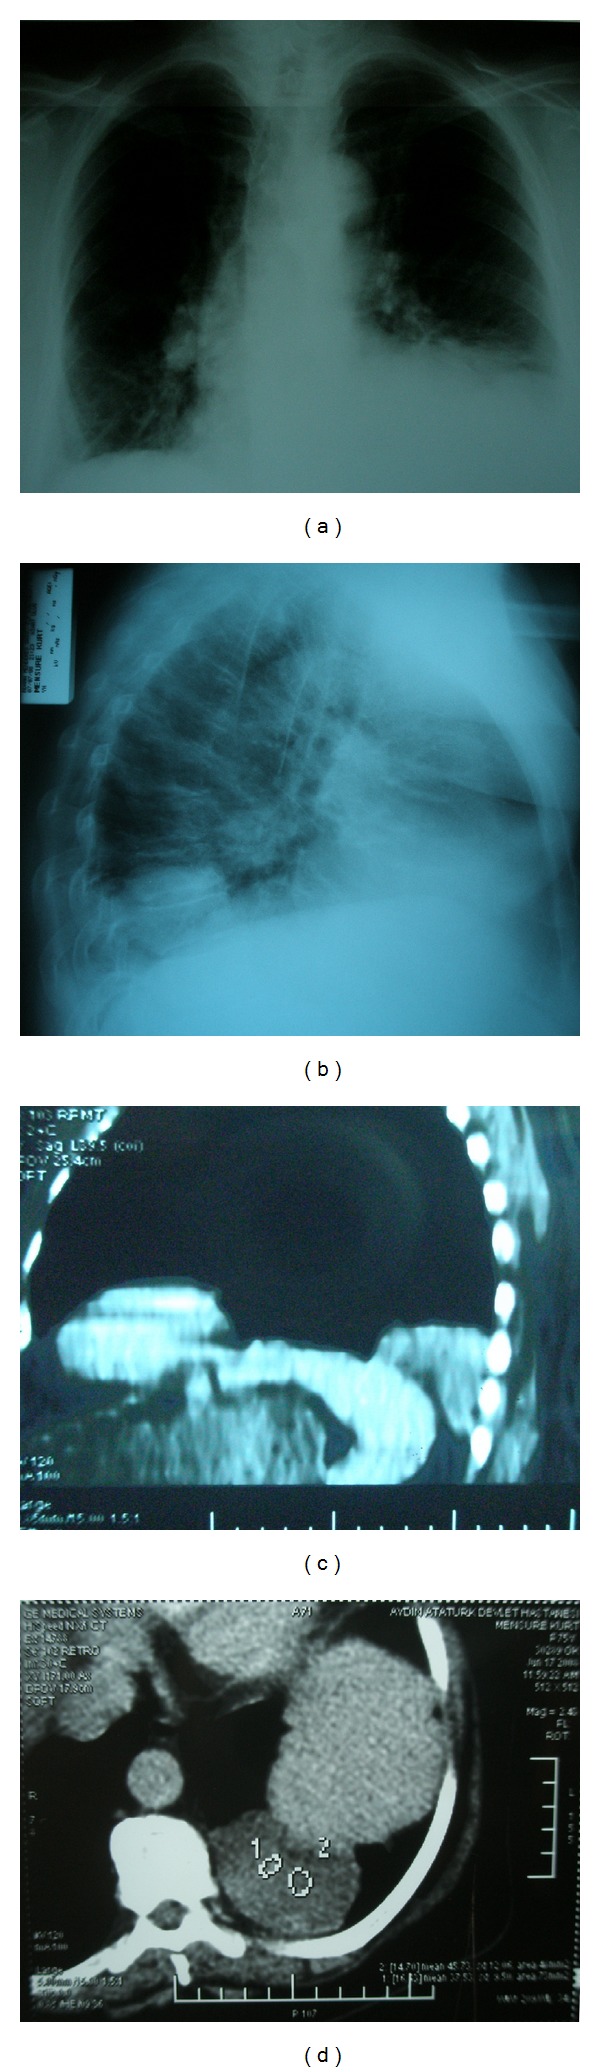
(a and b) Lesion can be seen in left hemithorax at inferior zone above diaphragm in chest radiographies (c and d) Thorax CT showing thatthe lesion has microcalcifications and close relationship with diaphragm and posterior costophrenic sinus.
